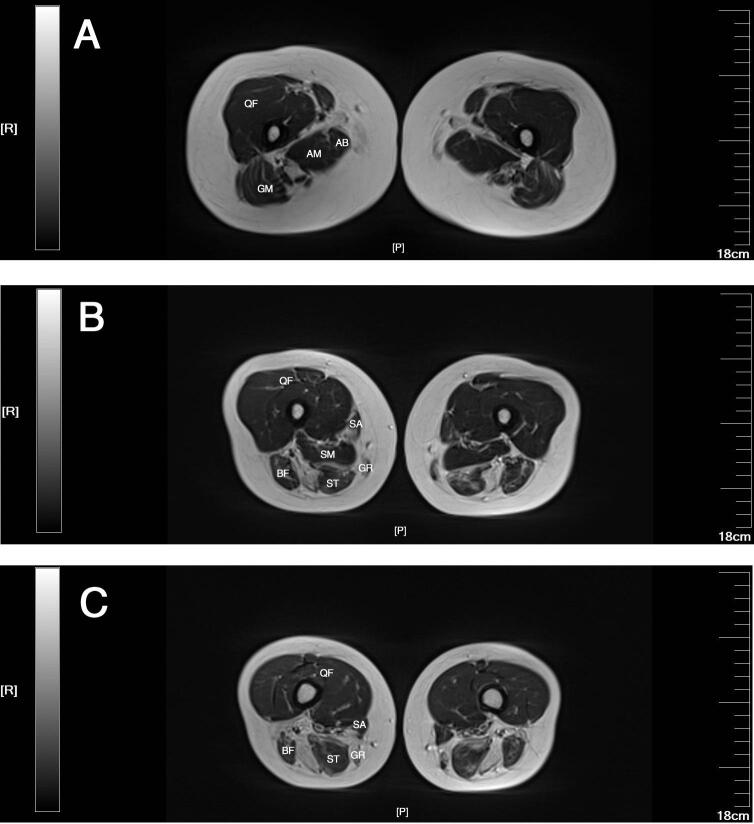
Axial T2-weighted magnetic resonance imaging (MRI) of the thighs at different planes, respectively the proximal (A), middle (B) and distal (C) planes. MRI of the adductor magnus (AM), adductor brevis (AB), gluteus maximus (GM), biceps femoris (BF), semitendinosus (ST), gracilis (GR), semimembranosus (SM) and sartorius (SA) showed different degrees of atrophy and fatty replacement. The quadriceps femoris (QF) were relatively preserved.
